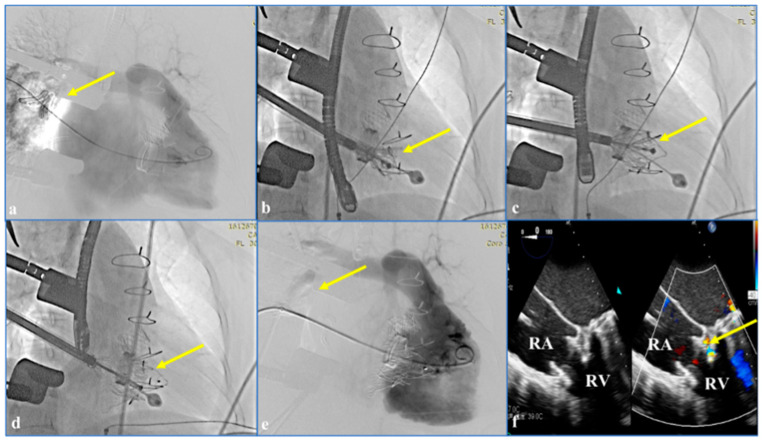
Intra- and postprocedural digital subtraction angiography and transesophageal echocardiography images showing the procedures. (a) Computed tomography angiography before the procedure showed massive regurgitation in the tricuspid valve; the arrow indicates the regurgitation. (b) The right atrium was incised and the delivery system was delivered into the right ventricle; the arrow points to the delivery system. (c) The anchor pin was positioned in the septum; the arrow points to the anchor pin. (d) The delivery system was separated from the tricuspid valve and withdrawn from the right atrium; the arrow points to the delivery system. (e) Computed tomography angiography shows no significant regurgitation; the arrow points to the tricuspid valve. (f) Transesophageal echocardiography displayed only mild TR; the arrow indicates the TR. Chinese words are temperature. RA: right atrium; RV: right ventricle.
